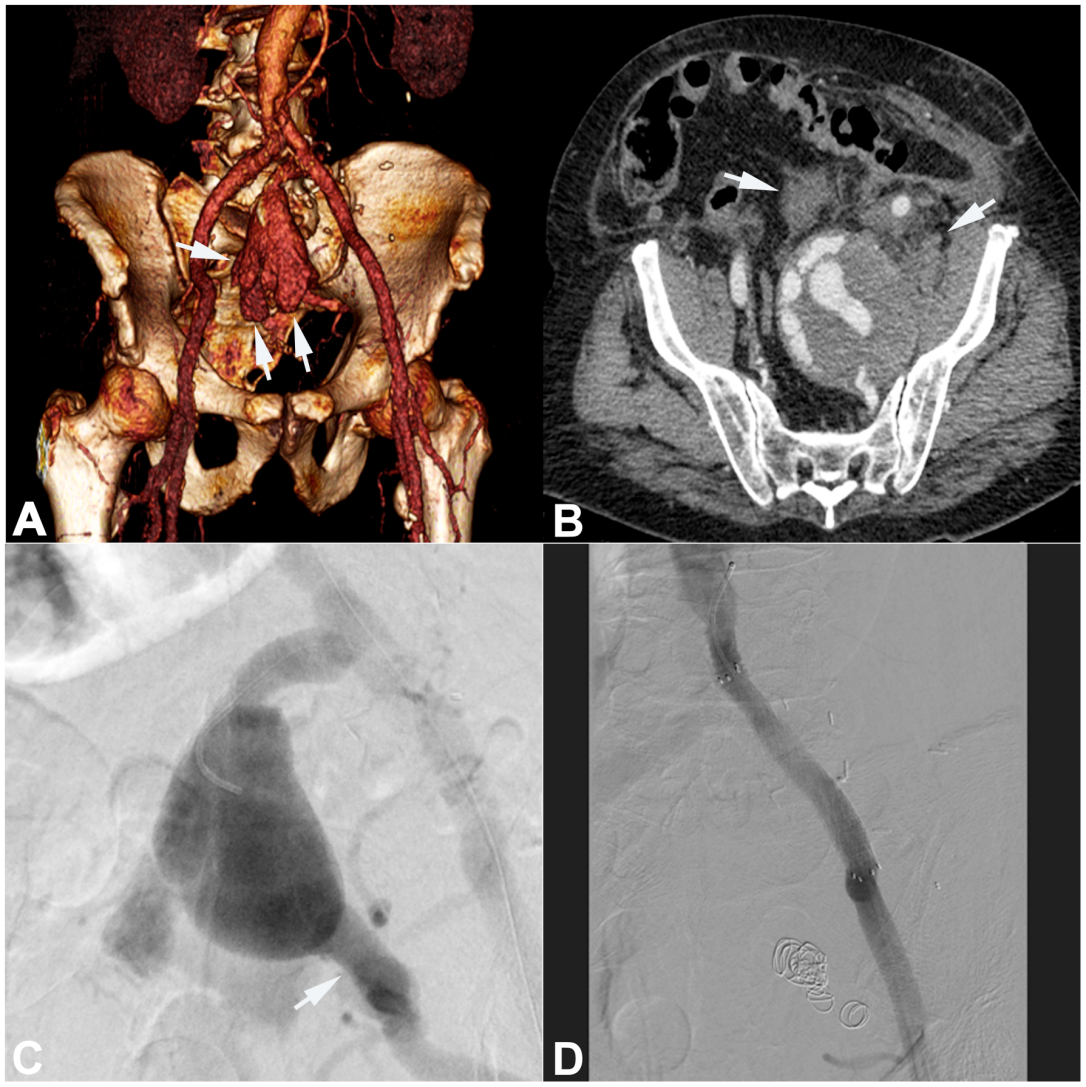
Successful stent graft placement of a ruptured IIA aneurysm in a 77-year-old man with acute abdominal pain in the left lower quadrant. A) Volume reconstruction (VR) shows a large left IIA aneurysm with the irregular boundaries (arrows) indicating a rupture. B) On axial CT scan the true size of the aneurysm is depicted with active bleeding within the thrombosed part and blood surrounding the aneurysm (arrows). C) Selective angiography shows a strong IIA aneurysm (arrow) with major side branches. D) Angiogram after stent graft placement and coiling of aneurysm shows complete exclusion of the aneurysm from blood flow.
